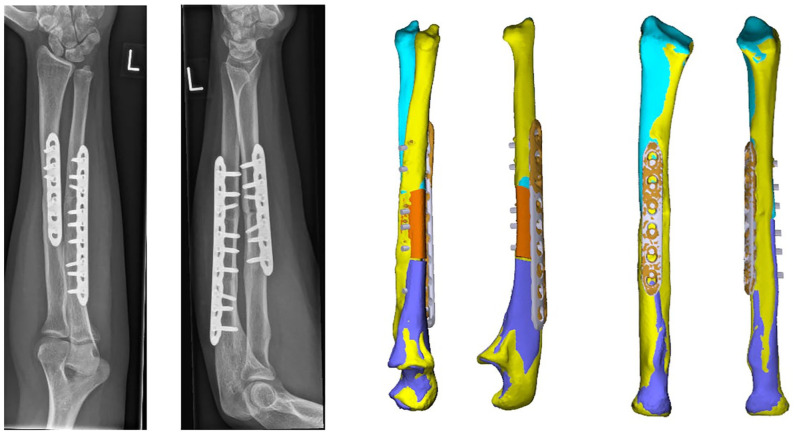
Posteroanterior and lateral radiographs and three-dimensional bone models with coronal and sagittal views of the left radius and ulna 1 year after surgery. *Note.* The planned distal part after the osteotomy is shown in blue, the middle part in orange (ulna only), and the proximal part in purple. Follow-up bones are superimposed in yellow. The planned position of the plate is shown in gray, and the actual position is in brown.
